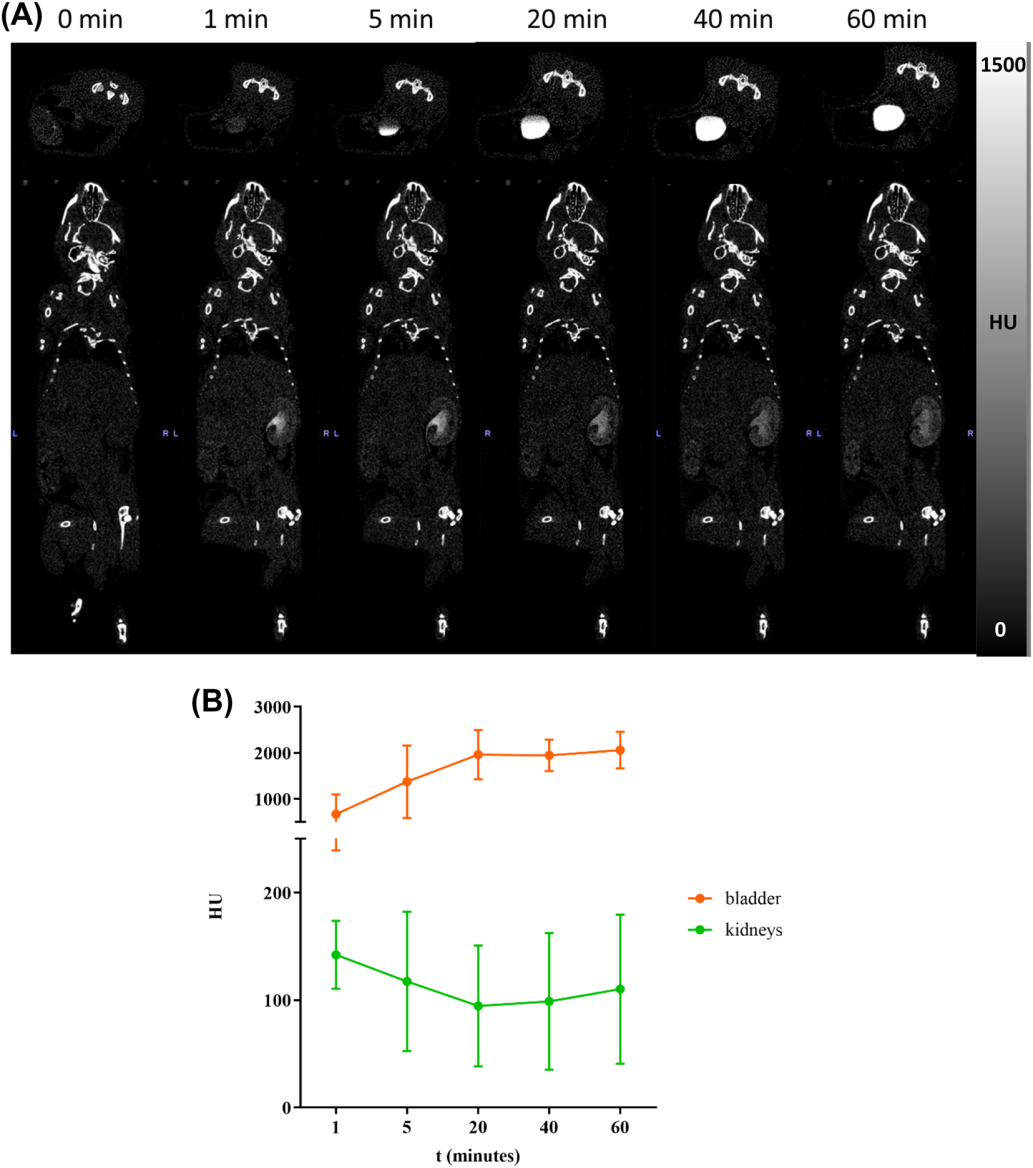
(A) In vivo CT imaging after i.v. administration of 1.2 mmol/kg of Bi-HPDO3A. Bladder and kidneys CT contrast are displayed. (B) VOIs analysis on bladder and kidneys for a dose of 1.2 mmol/kg of Bi-HPDO3A.
